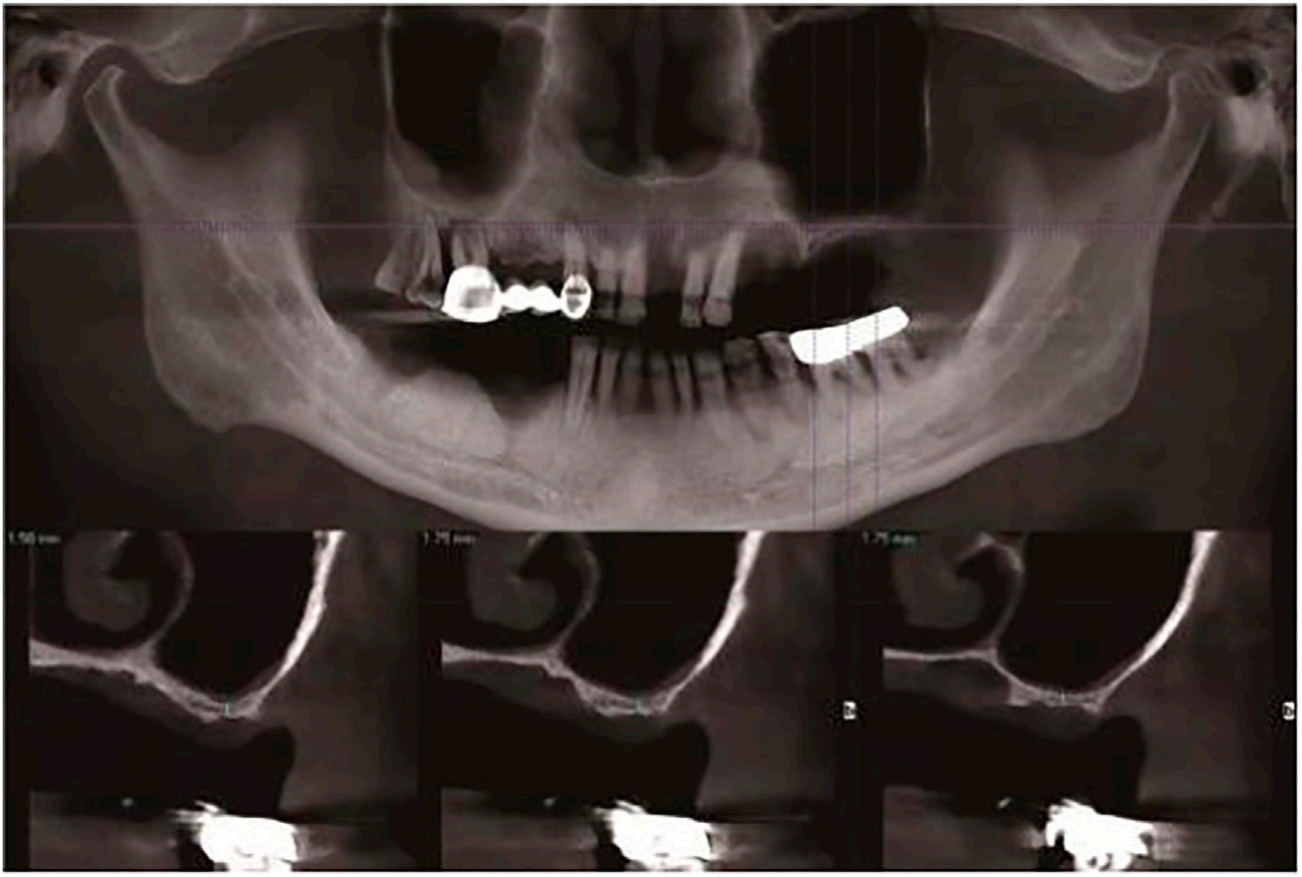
Measurements of the CBCT for preoperative evaluation.
